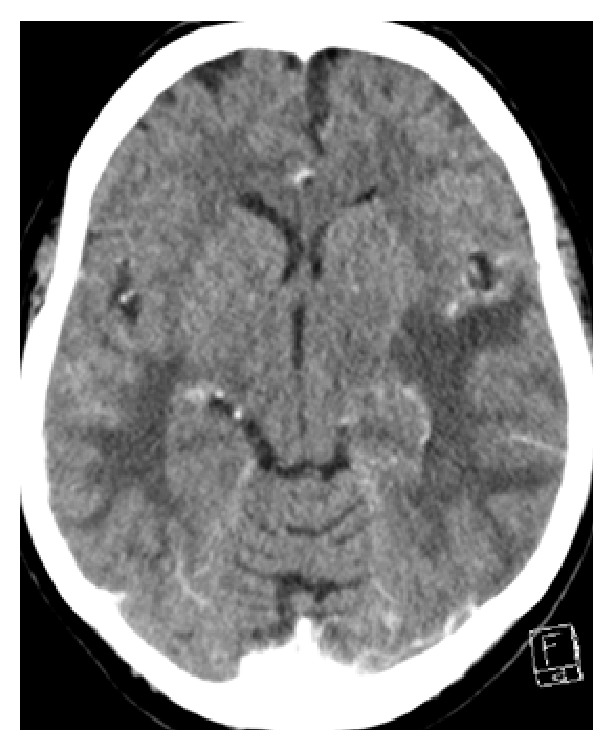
Contrast enhanced CT brain showing bilateral vasogenic edema of the parietal and temporal lobes.
